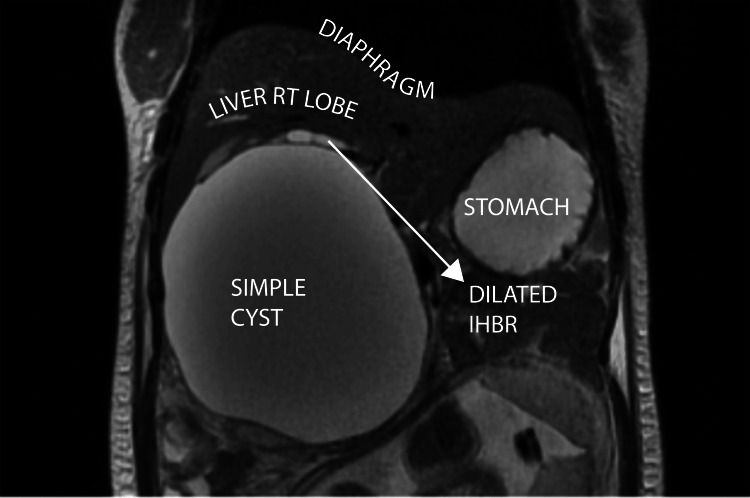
Coronal T2-weighted MRI - image 1 The image shows a T2 hyperintense cystic lesion measuring 14.2 x 12 x 12.8 cm. It is indenting the right lobe of the liver (superiorly) MRI: magnetic resonance imaging
